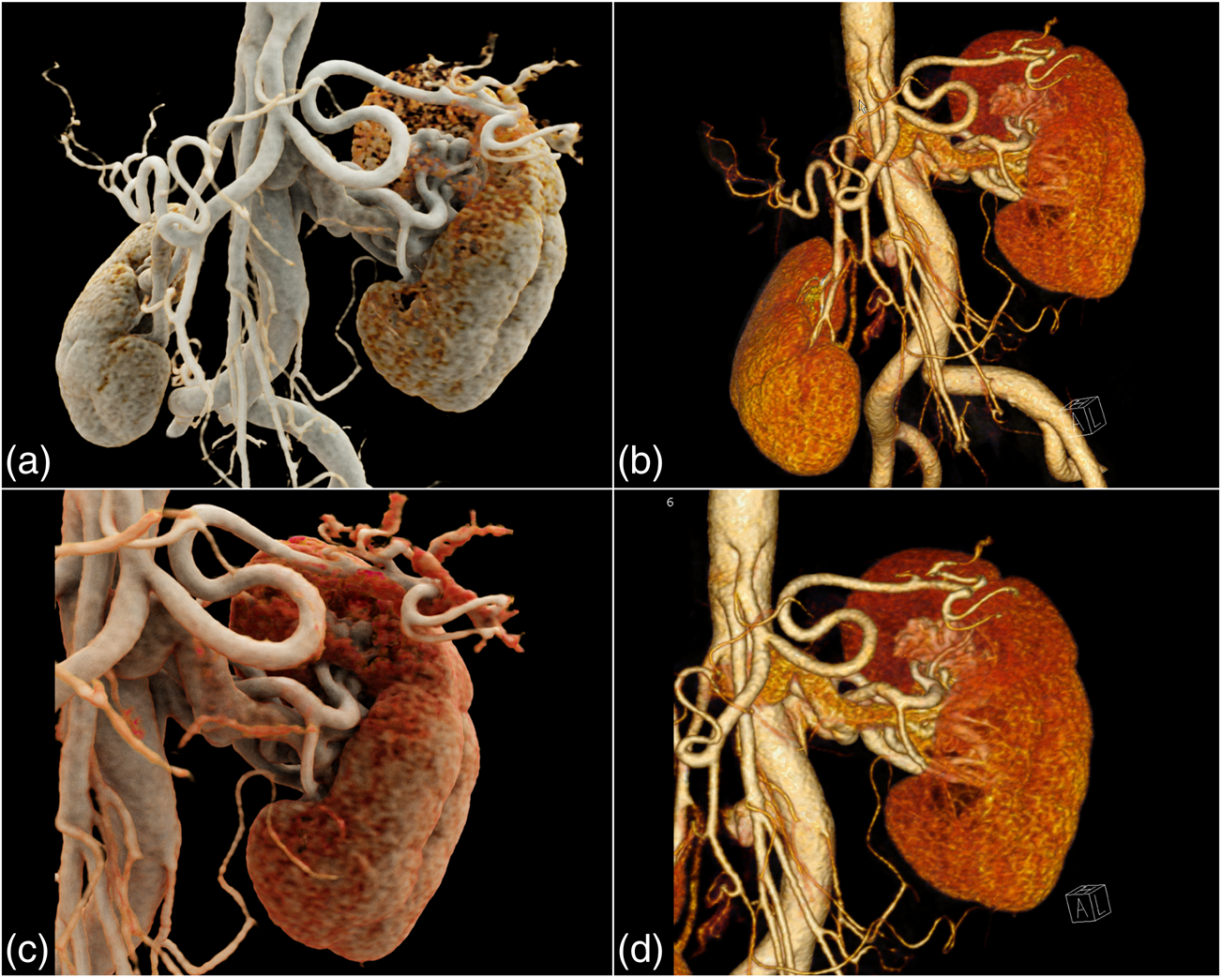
Contrast-enhanced 3-D CT images of an arteriovenous malformation (AVM) in the upper pole of the left kidney. CR (a) and VR (b) images and close-up view (c, d) images allow for a more detailed depiction of the AVM. The tortuous vessels at the upper kidney pole are visualized with a more realistic representation on CR than on VR images
